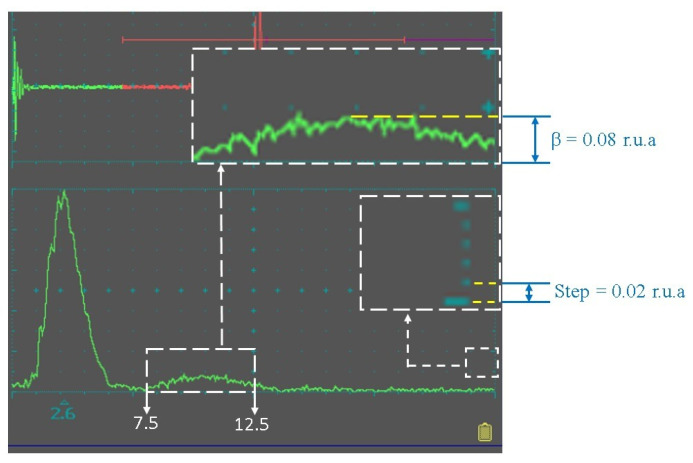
Determination of the third harmonic amplitude of the bottom signal using the UCD-60N ultrasonic flaw detector.
